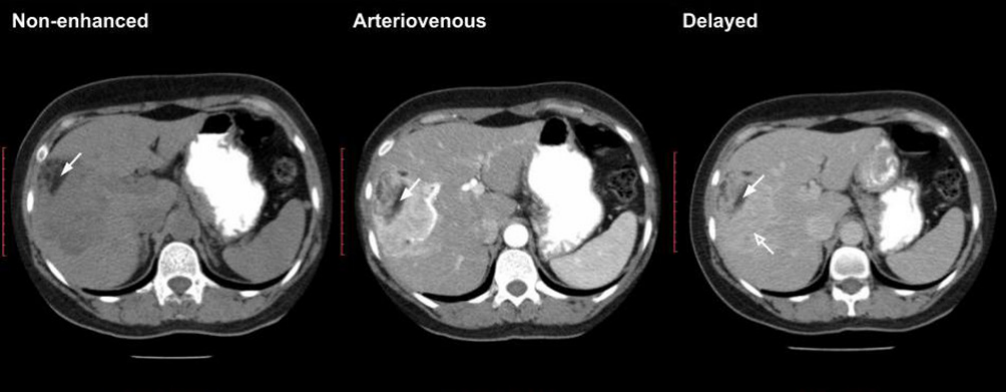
CT with intravenous and oral contrast enhancement (non-enhanced, arteriovenous, delayed phases). Large highly vascularized soft-tissue tumour with peripheral macroscopic fatty components (white arrows) located in right hepatic lobe. Some contrast material retention is noted in the delayed phase (open white arrow).
